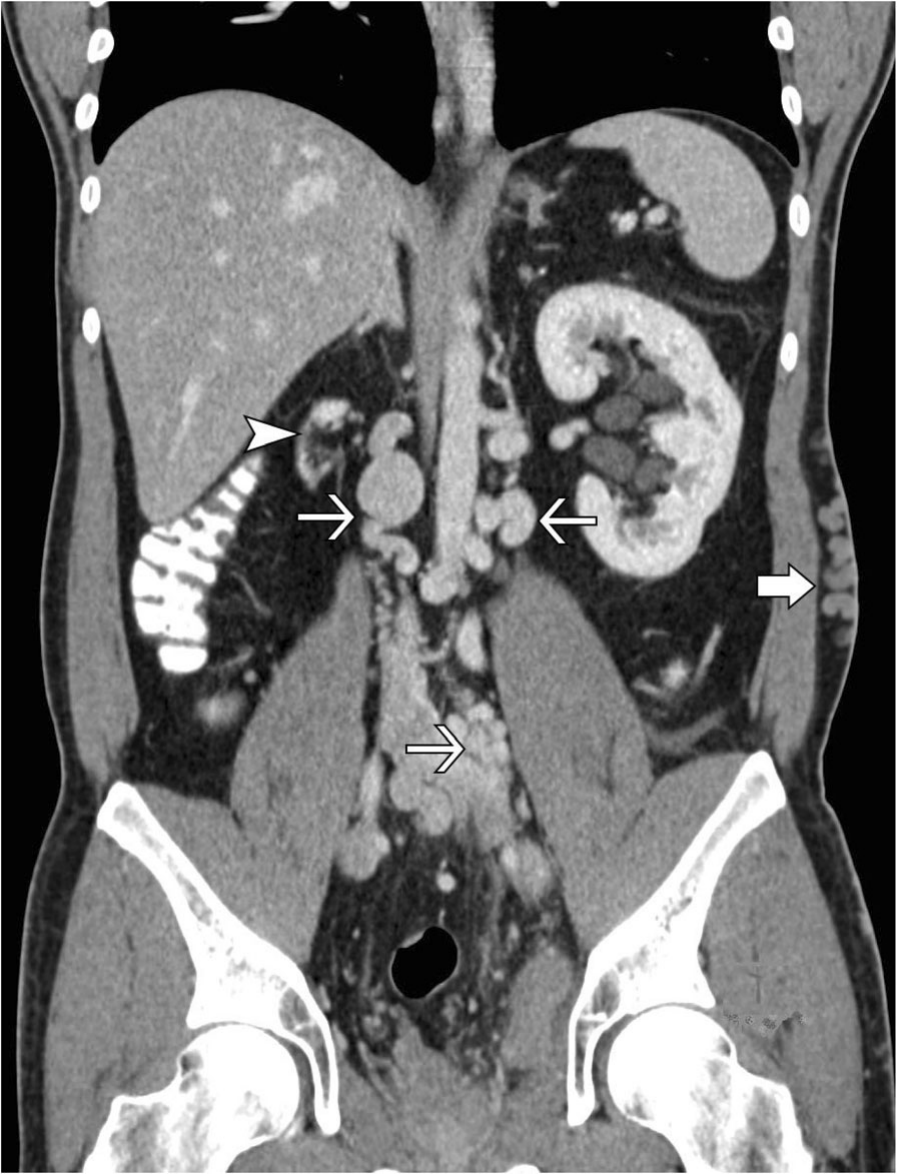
Coronary abdominal CT Scan. CT shows shrunken right kidney (arrowhead), extensive venous collateral system (thin arrows) and subcutaneous collateral vessels (thick arrow)
